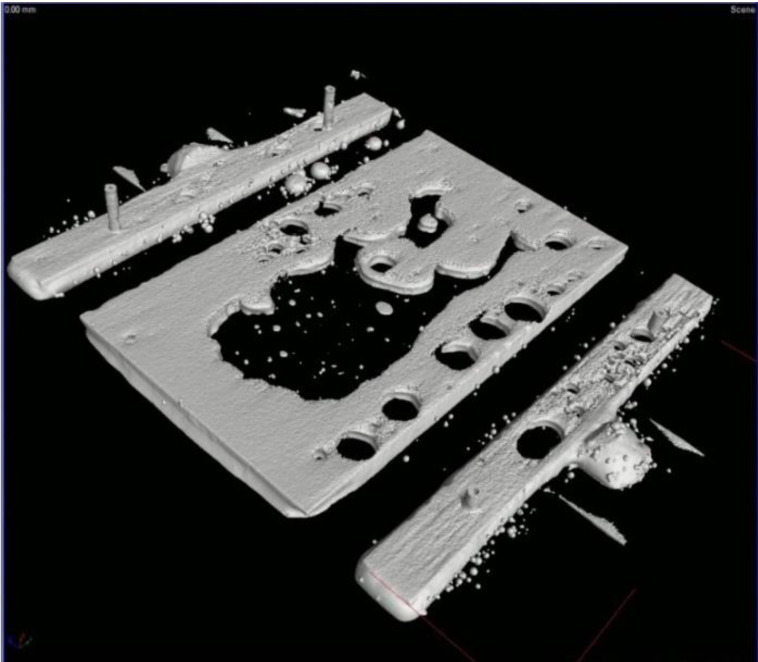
Three-dimensional (3D) CT image of a SACX0307-ZnO-(50 nm) solder joint.
